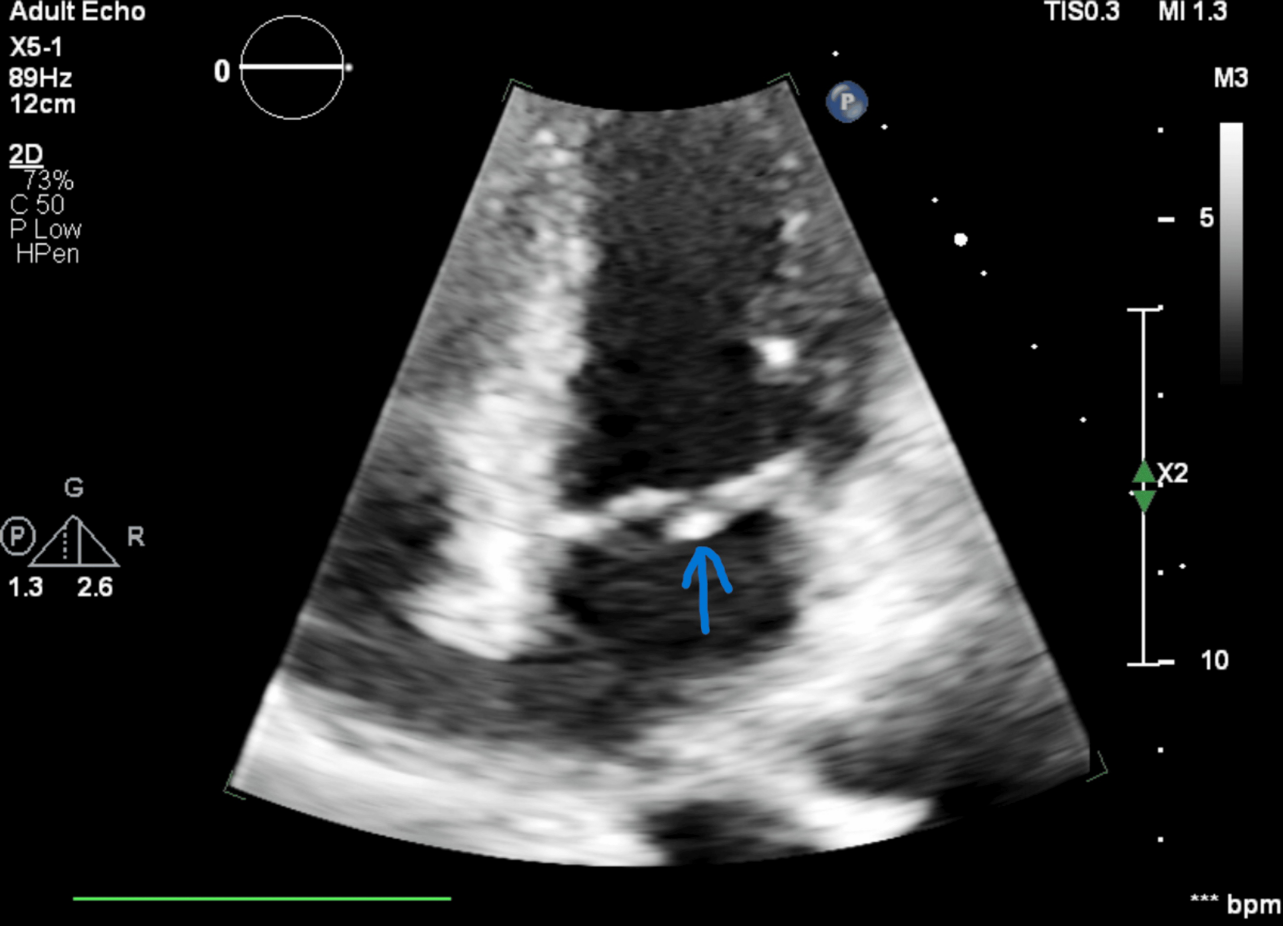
Transthoracic echocardiography Two-dimensional transthoracic echocardiographic image demonstrating a rounded, well-defined echogenic mass attached to the posterior leaflet of the mitral valve (arrow), raising suspicion of a valvular tumor.
